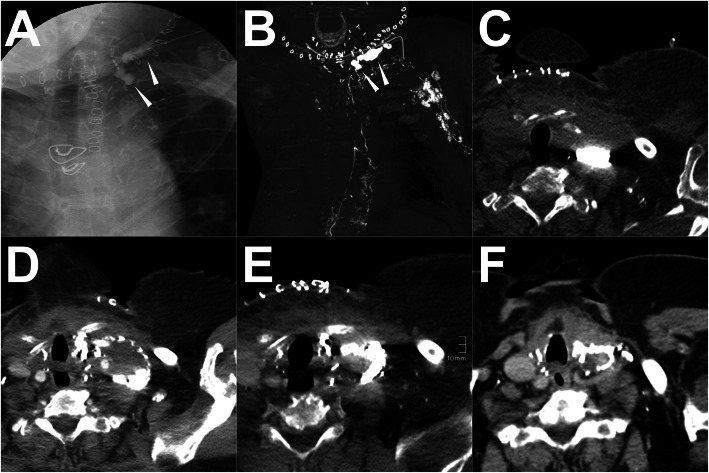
A patient underwent no interventions after iodized oil-based LAG. Note: Both the conventional iodized oil-based LAG (a) and post-LAG CT (b) reveal the definite rupture of the thoracic duct (white arrowhead) in the neck. In addition, the reflux of lipiodol to the left axillary and upper arm was observed which indicates the disruption of the end of the thoracic duct (b). In the axial post-LAG CT image, the fluid accumulation with the iodized oil extravasation is noticed (c). No other intervention was carried out in this patient except for the conventional iodized oil-based LAG treatment. However, with time (6d, 11d) (d-e), the fluid accumulation gradually reduced and the drainage eventually ceased on the 11th day. Two (2) months after LAG, the recheck of the CT scan shows no recurrence of the LF (f). Abbreviations: LAG – lymphangiography; post-LAG CT – post-lymphangiographic computed tomography
